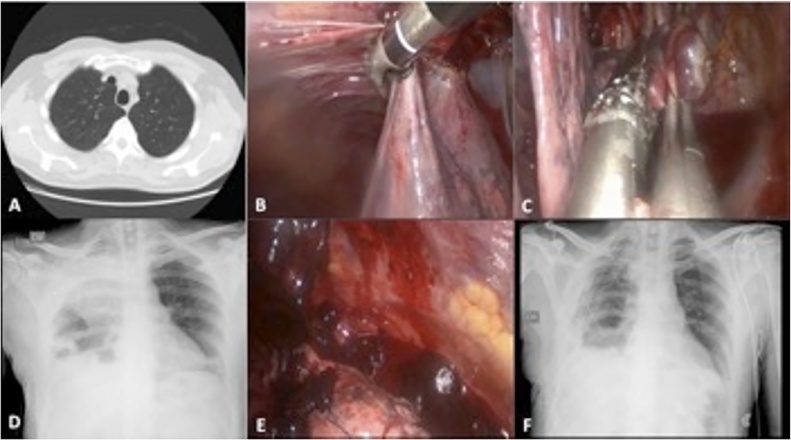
Pre operative CT-scan showed an apical bullae in the right upper lobe, in absence of pneumothorax. (A) Partial lysis of pleural adhesions and apical bullectomy were done using vessel sealing and stapling devices. (B–C). A 24 h post operative chest X-ray showed a right pulmonary radiopacity suspected for hemothorax. The presence of clots were confirmed in course of redo-VATS. (D–E) Post operative chest X-ray showed full re-expansion of the pulmonary parenchyma following to complete pleurolysis in redo-VATS. (F).
